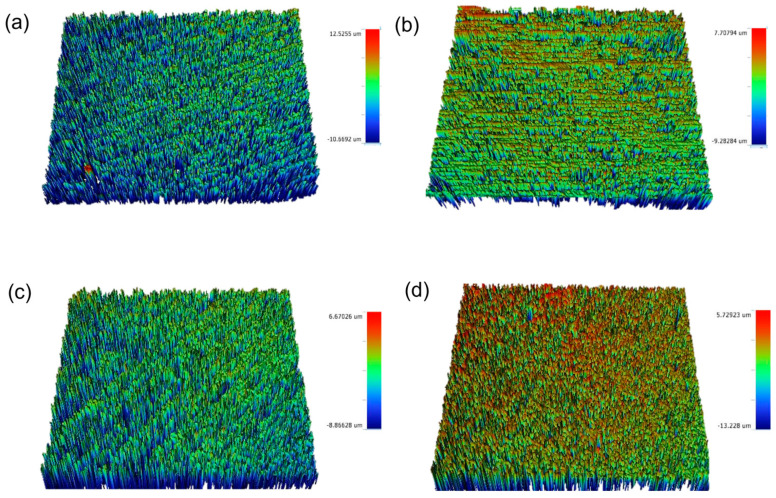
Surface roughness (Ra) of ultrasonic scaling of each: (a) CD, (b) CT, (c) IPE, and (d) IPS. CD; zirconia-reinforced lithium silicate (Celtra Duo). CT; advanced lithium disilicate (CEREC Tessera). IPE; lithium disilicate (IPS Emax CAD). IPS; leucite-reinforced (IPS Empress CAD).
